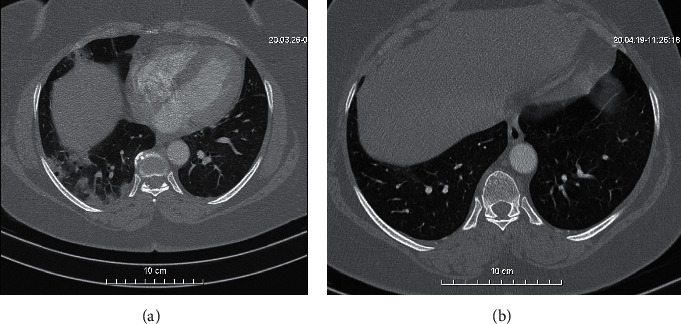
(a) Chest computed tomography (CT) scans (transverse plane) of the patient 2: multifocal nodular condensations with halo sign (peripheral frosted glass) under right posterobasal and anterobasal pleura. (b) Chest computed tomography (CT) scans (transverse plane) control of the patient 2: favorable evolution with disappearance of nodular condensations under right posterobasal pleura.
